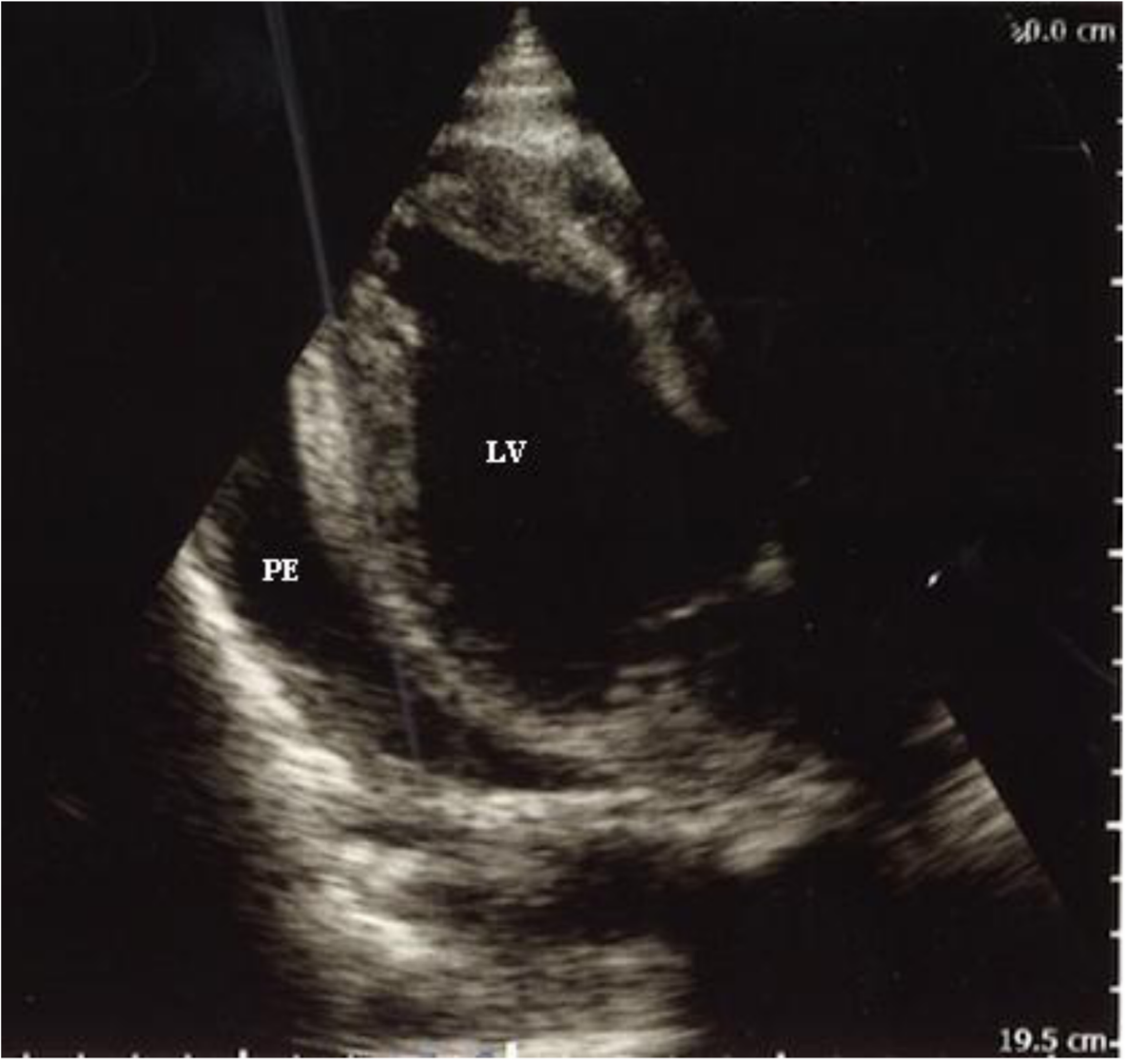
Echocardiography (parasternal long axis view) prior to the initial pericardiocentesis in rural North Greenland. The pericardial effusion (PE) is seen. LV, left ventricle.
